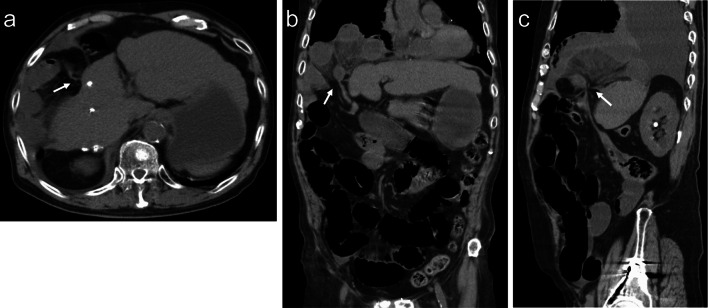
CT image. a Axial view, b coronal view, c sagittal view. The small intestine herniated through the right diaphragm with intestinal expansion (arrow). Pleural effusion was observed in the right chest cavity
